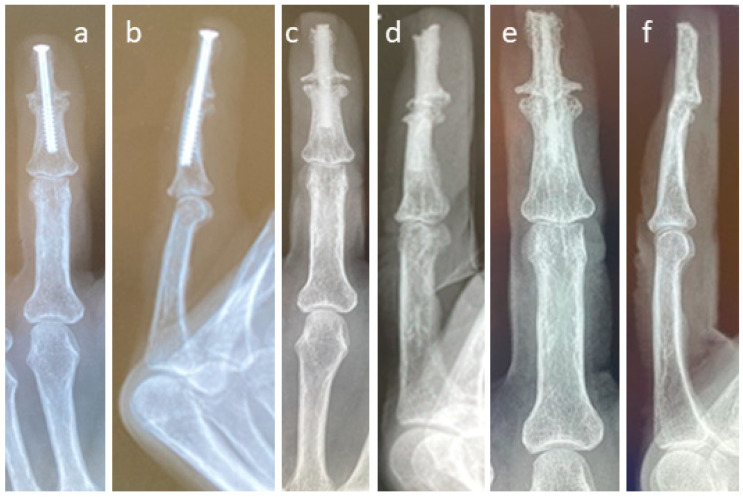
The revision after a failed arthrodesis of the middle finger with a metal screw. (a,b): The X-ray after the first treatment. Even hardware removal did not lead to union 31 months after surgery. (c,d): For the revision, one Shark Screw^®^ was placed, and union was obtained after 26 weeks. (e,f): 36 months after surgery, the Shark Screw^®^ is nearly totally remodelled.
